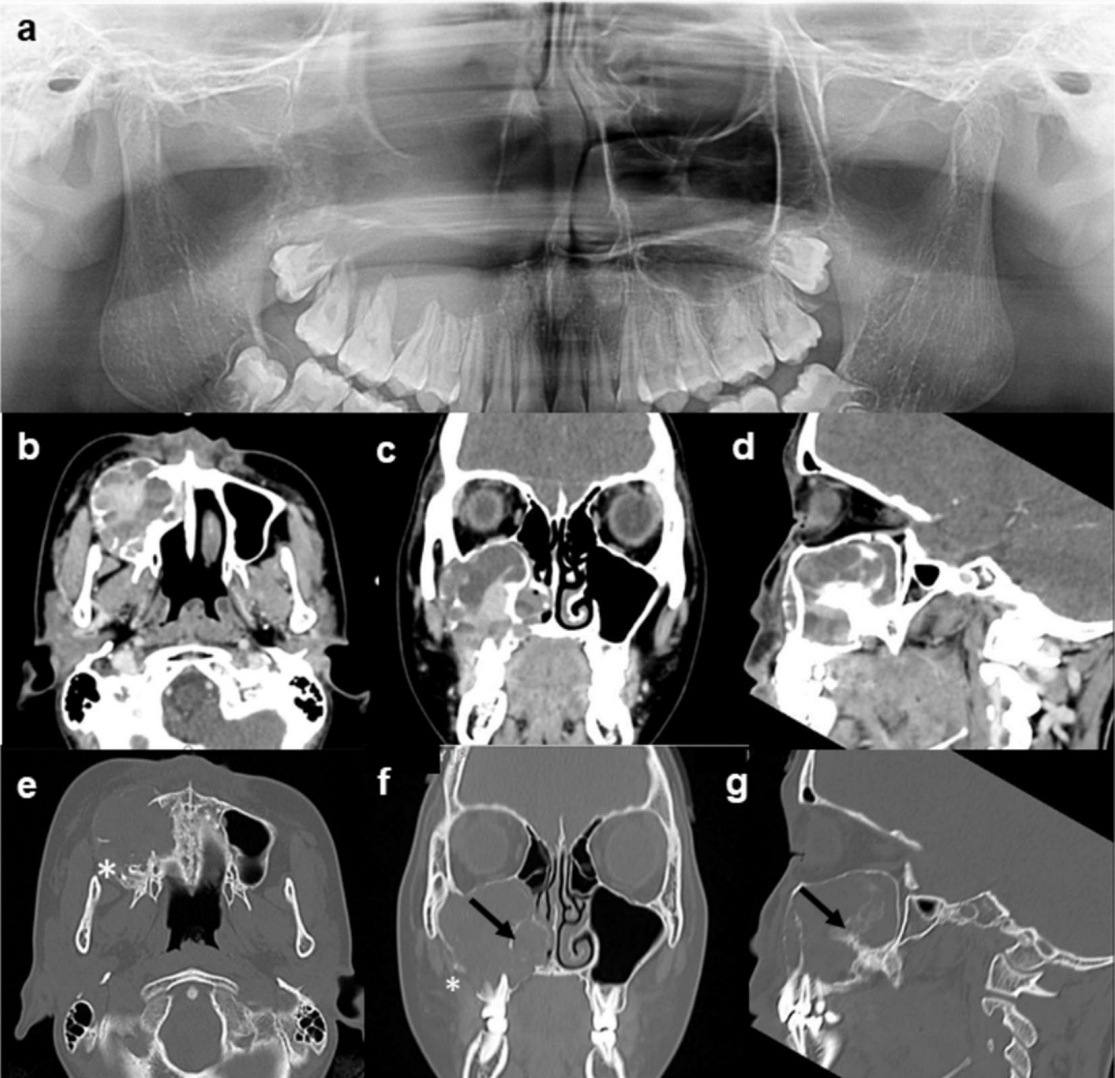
Radiographs of case 5. A cropped panoramic image (a), multidetector computed tomography (MDCT) images with soft tissue window (b-d) and MDCT images with bone window (e-g) show an expansile mass with heterogeneous enhancement at the right maxilla occupying the right maxillary sinus, right middle and inferior turbinates with expansion of the maxillary sinus walls and area of cortex perforation (*). The lesion shows scalloped border with internal septum (arrows)
